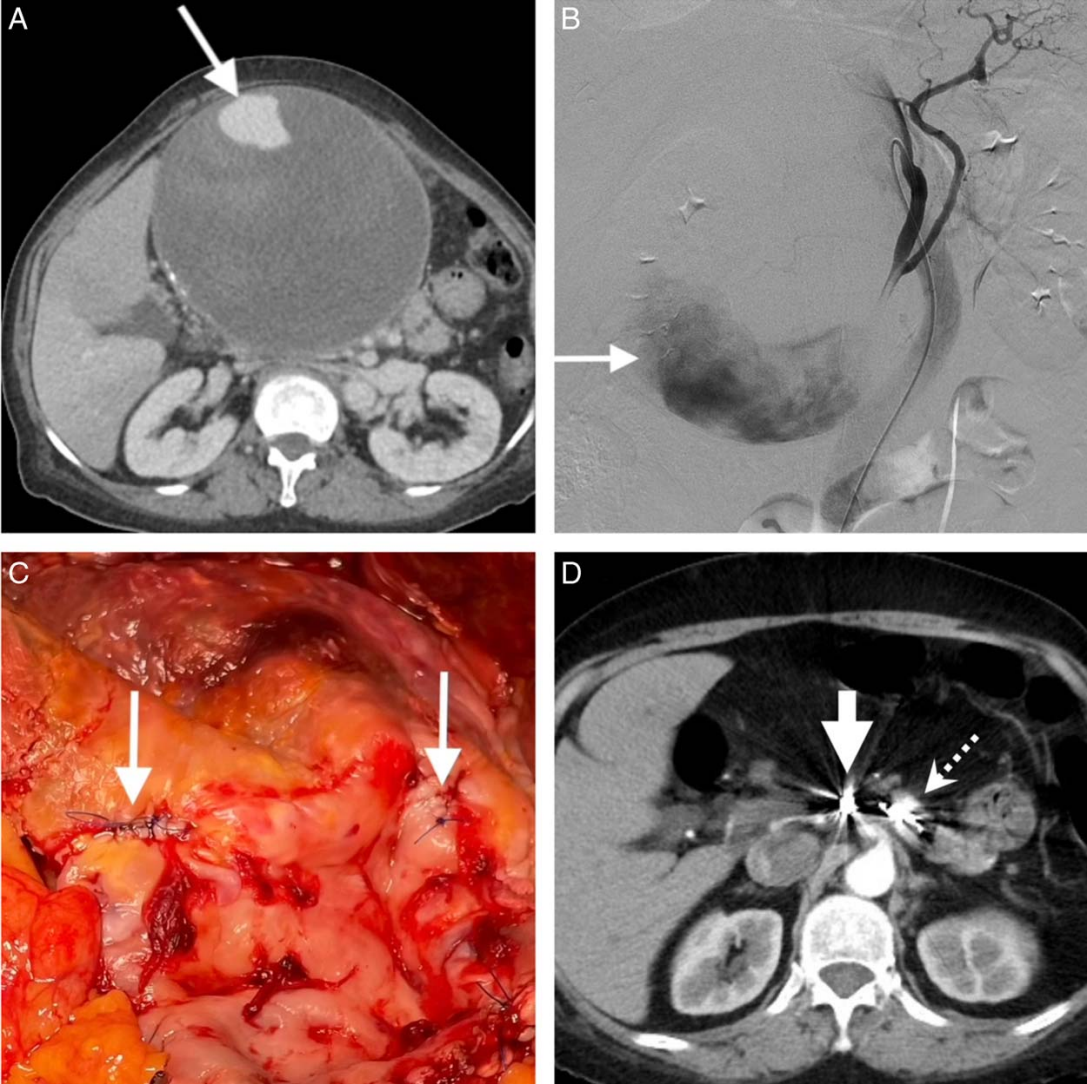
Dorsal pancreatic artery false aneurysm (A) Abdominal CT showed a peripancreatic false aneurysm about 160 mm (arrow) with intraluminal thrombosis. (B) Angiography confirmed an aneurysm from the dorsal pancreatic artery (arrow), so we performed coil angioembolization via the splenic artery but got coil migration to the distal splenic artery. (C) We offered a salvage surgery for partial aneurysmectomy and direct suturing of the feeding vessels’ orifice from the aneurysm’s inner lumen (arrow). (D) Postoperative CT showed complete resolution of the giant aneurysm with the remaining coil at the dorsal pancreatic artery (large arrow) and the migrated coil at the distal splenic artery (small arrow).
